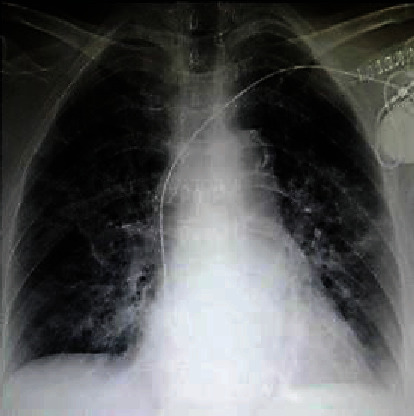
The chest X-ray of the COVID-19 patient after the pPM implantation.
